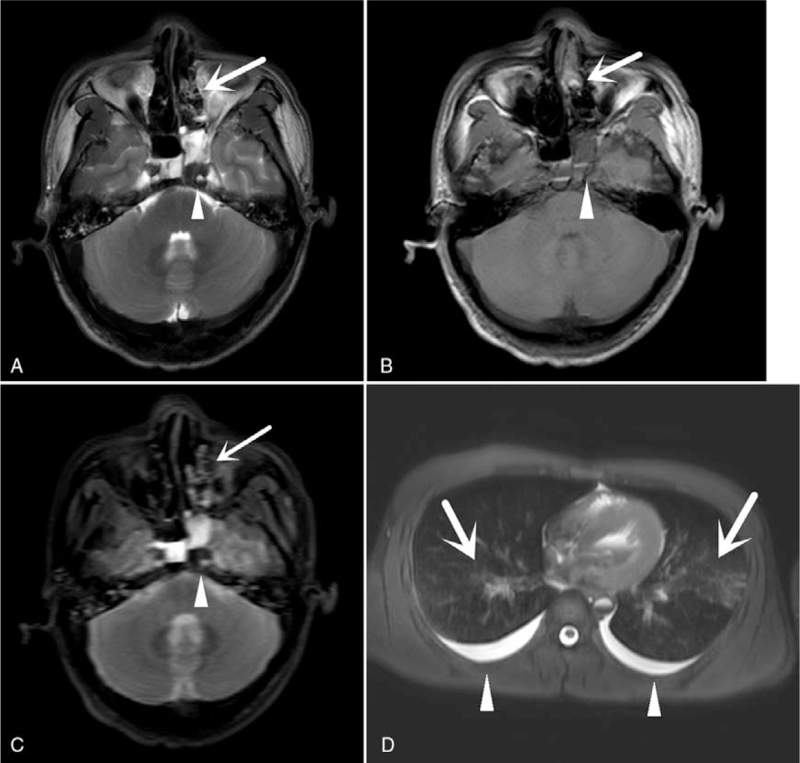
PMMRI findings. (A–C) T2WI, T1WI, and T2W FLAIR images showing hemorrhage in the sphenoid sinus (white arrowhead) and nasal cavity (white arrow). (D) T2WI showing a small amount of pleural effusion on both sides (white arrowhead) and some congestion of both lungs (white arrow).
